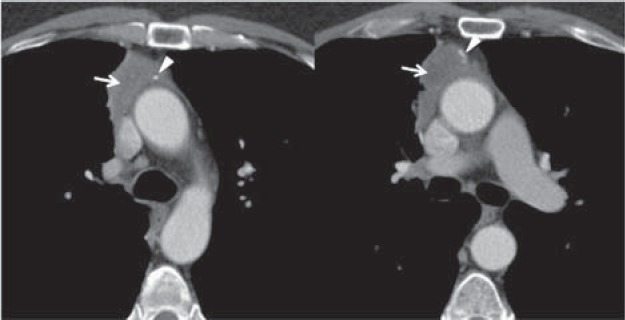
52-y/o male with myasthenia gravis; Contrast-enhanced CT scan shows lobulated contour mass at the anterior mediastinum with heterogeneous enhancement, areas of decreased attenuation (arrows) and calcified foci (arrowheads); pathologic diagnosis – type B3 thymoma.
